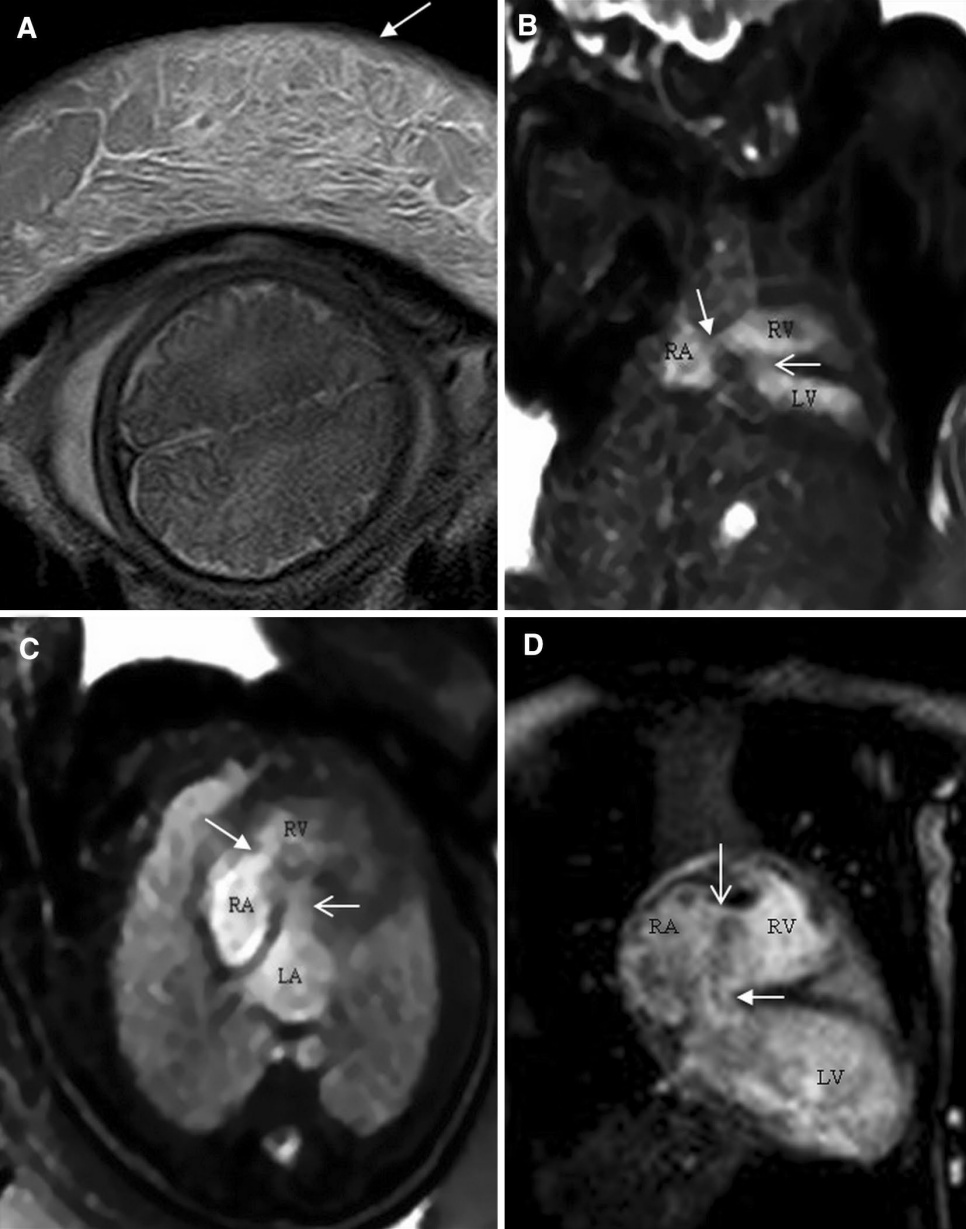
A 34-week-old foetus with a criss-cross heart. A 34-week-old foetus had a echocardiography at 22 weeks of gestation that suggested the presence of transposition of the great arteries (D-TGA) and ventricular septal defect (VSD). Follow up foetal echocardiography at 34 weeks of gestation was limited because of increasing maternal subcutaneous oedema and obesity. An axial SSTSE image of the maternal abdomen demonstrating oedema of the subcutaneous soft tissues (A, arrow). SSFP coronal and axial view images showing the right ventricle located superiorly (B, RV), the horizontal ventricular septum with a VSD (B, open arrow), the right atrium (B,C RA) connected to the right ventricle (B,C RV) in a right-to-left direction (B,C arrow), and the left atrium (LA) connected to the left ventricle (LV) in a posteroanterior direction (C, open arrow). A postnatal enhanced cardiac MR image showing a criss-cross heart (same as the prenatal cardiac MRI). The right ventricle was located superiorly (D, RV), the horizontal ventricular septum with a VSD (D, arrow), the right atrium (D, RA) was connected to the right ventricle (D, RV) in a right-to-left direction (D, open arrow). The left atrium (LA) was connected to the left ventricle (LV) in a posteroanterior direction.
